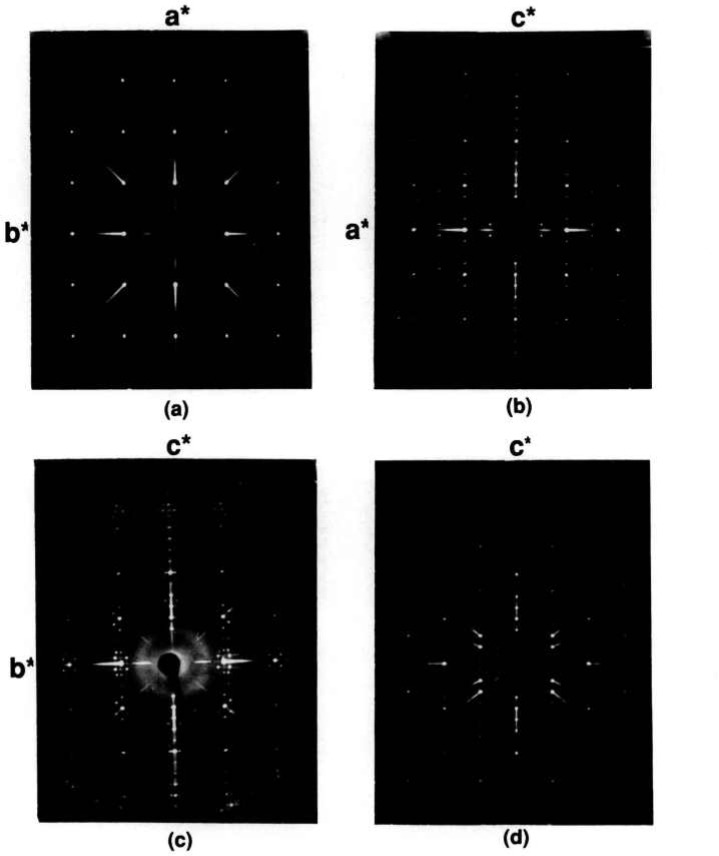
X-ray precession photographs of an orthorhorabic/incommensurate Raveau solid solution phase that was grown in 1:1 NaF:KF flux. Original composition=Sr_2_Bi_2_CuO_6_ (a) *hk0*, (b) *h0l*, (c) *0kl* and (d) *hhl.*
